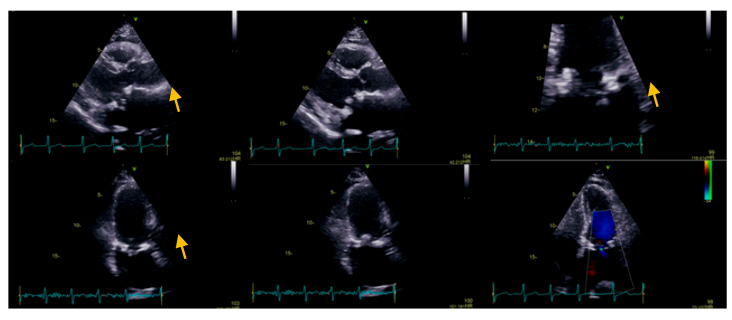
Transthoracic echocardiography: parasternal long axis view, the zoomed image on mitral valve (apical four-chamber view), and the apical two-chambers view, the yellow arrow showing a mobile, isoechoic, homogeneous filiform mass of 10 mm, attached to the mitral prosthetic valve, with minor intra-prosthetic regurgitation.
